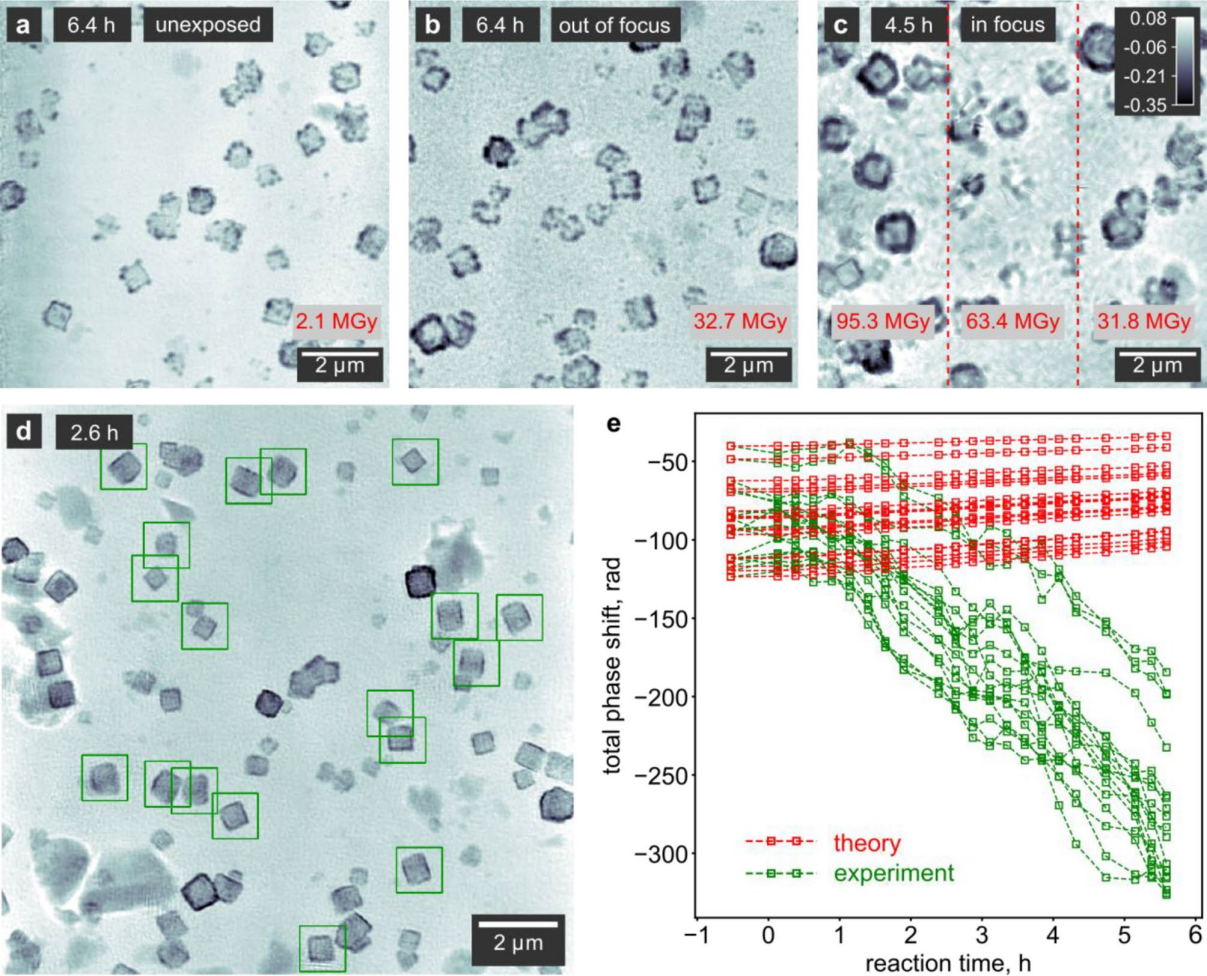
Quantification of radiation damage. (a,b) In situ ptychographic reconstructions during the GRR obtained at the same reaction time, showing (a) a region previously unexposed to X-rays and (b) a region continuously exposed for the preceding 6.4 h in the defocus of the X-ray beam. (c) Ptychographic reconstruction of a GRR exposed in the X-ray focus with a shifting field of view. The gray scale indicates the phase shift in radian. (d) Ptychographic reconstruction highlighting a number of areas around individual particles used for the quantitative phase analysis. The reconstructed pixel size is 14.51 nm. (e) Expected evolution of the total phase shift of the particles (red) highlighted in (d), assuming a full conversion from Cu_2_O to Au, compared to the measured phase shift (green). We applied a moving average with a window size of 3 data points. A larger negative phase shift indicates more material. Due to the unknown amounts of Cu_2_O and Au in the sample, we can not convert the measured phase shift into an electron count (see Supplementary Note 5 for details).
